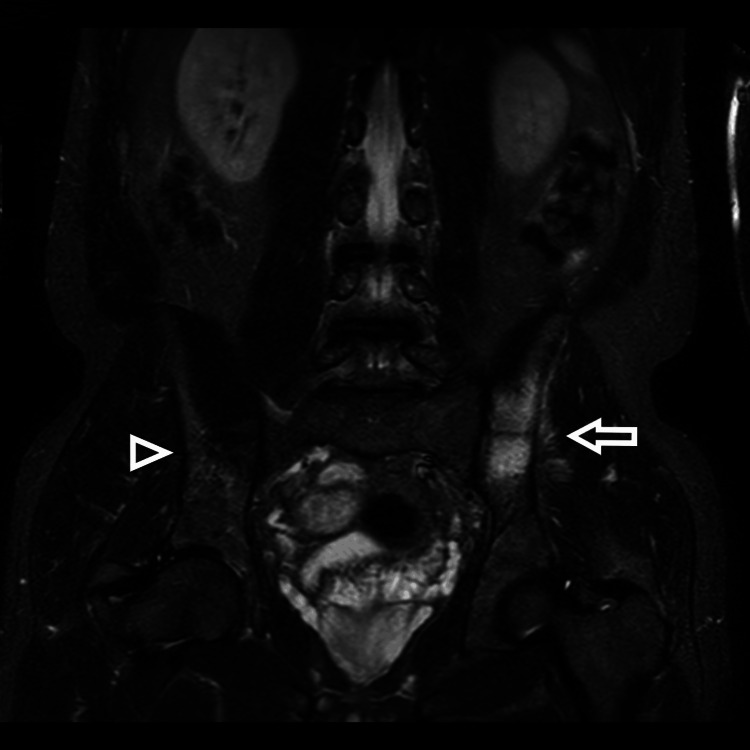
Second MRI coronal view with STIR TSE, revealed a second symmetric contralateral ilium fracture (arrow) with partial regression of oedema around the initial right ilium fracture (arrowhead). STIR-Short Tau Inversion Recovery, TSE-Turbo Spin Echo.
